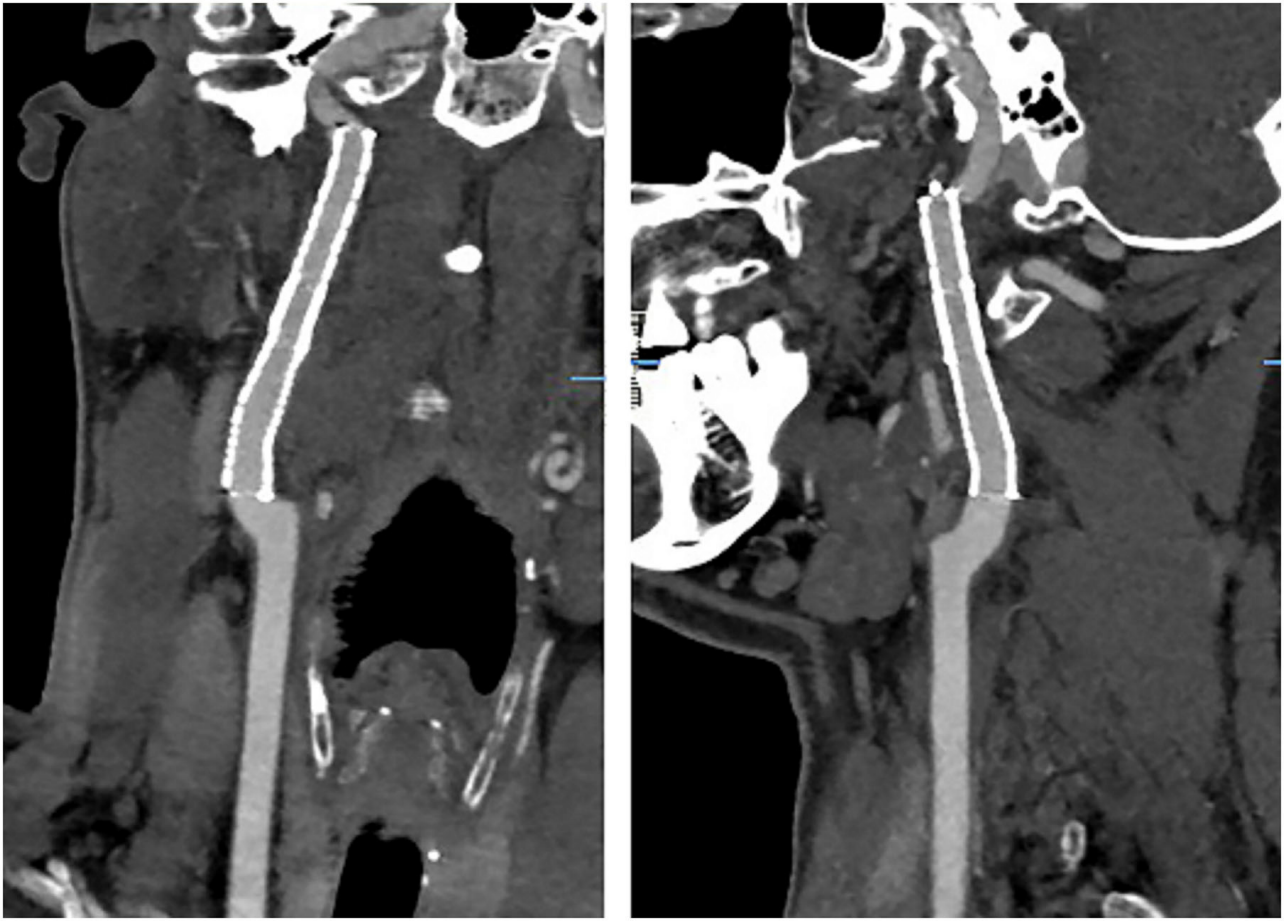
The CTA revealed that the lumen was unobstructed at 3 months after stenting.
